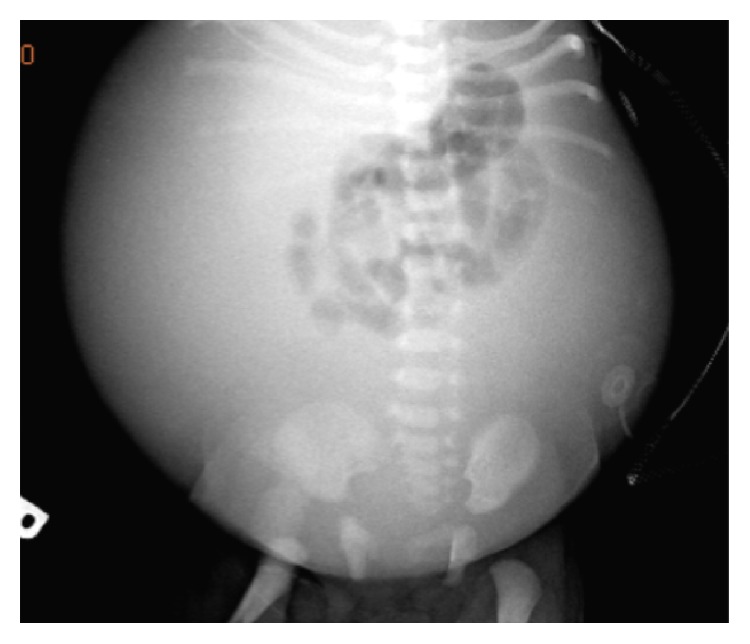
Abdominal X-ray, day of life 1: distended abdomen with centralization of bowel loops showing massive ascites.
